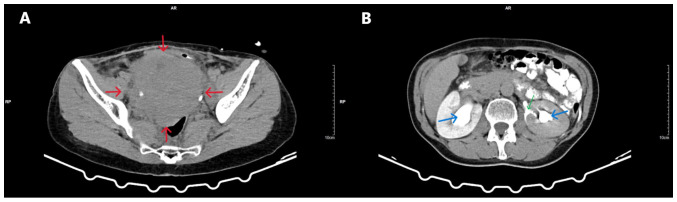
Computed tomography imaging of a giant endocervical fibroid associated with bilateral ureterohydronephrosis and retroperitoneal perinephric urinoma. (A) A large, solid, hyperechoic mass with clear borders (red arrows) occupying the pelvis and compressing adjacent anatomical structures. (B) Bilateral dilation of the pelvicalyceal system (blue arrows) and extravasation of contrast material into the left kidney, corresponding to a retroperitoneal perinephric urinoma (green arrow).
